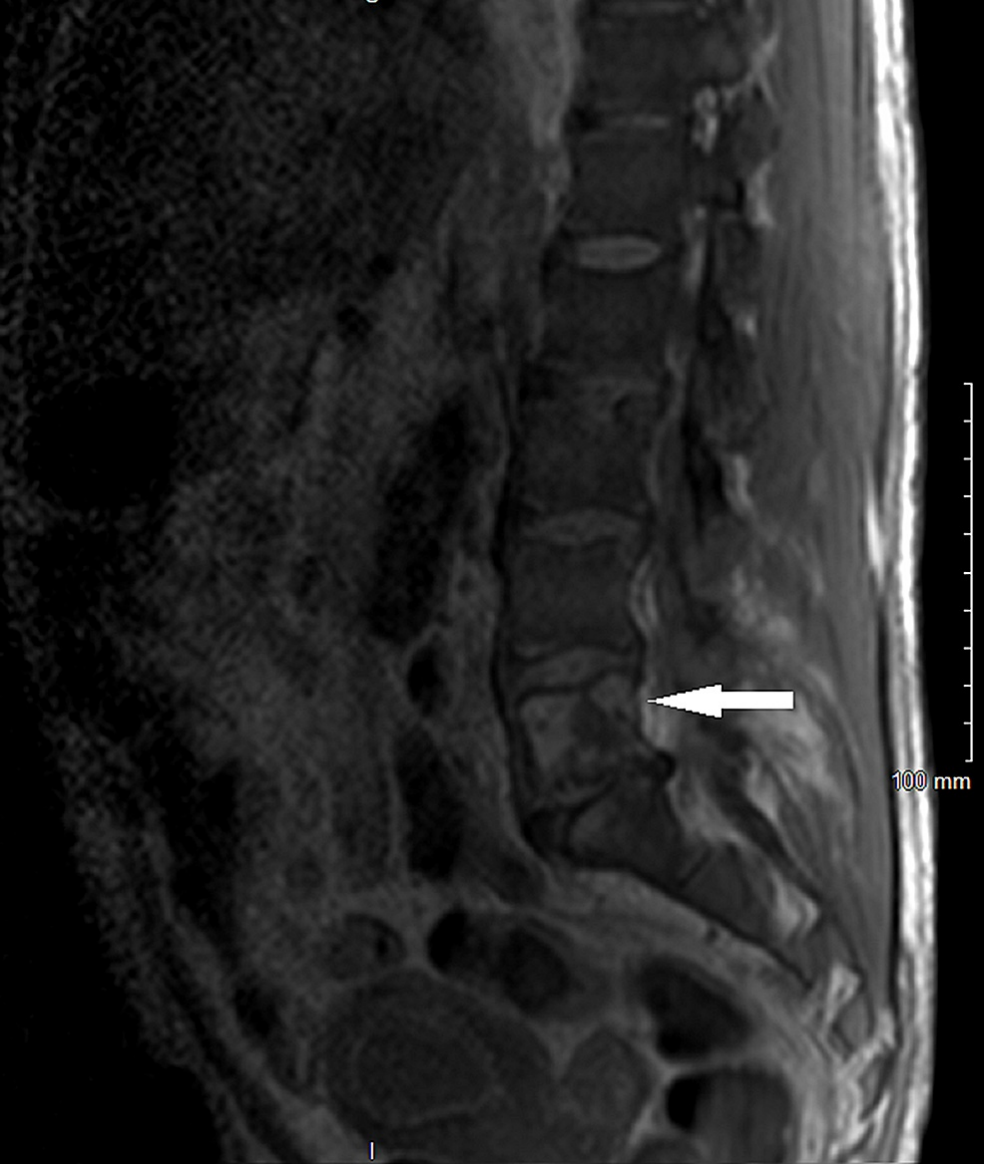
MRI of the lumbar spine without contrast showing the L5 lytic lesion.
